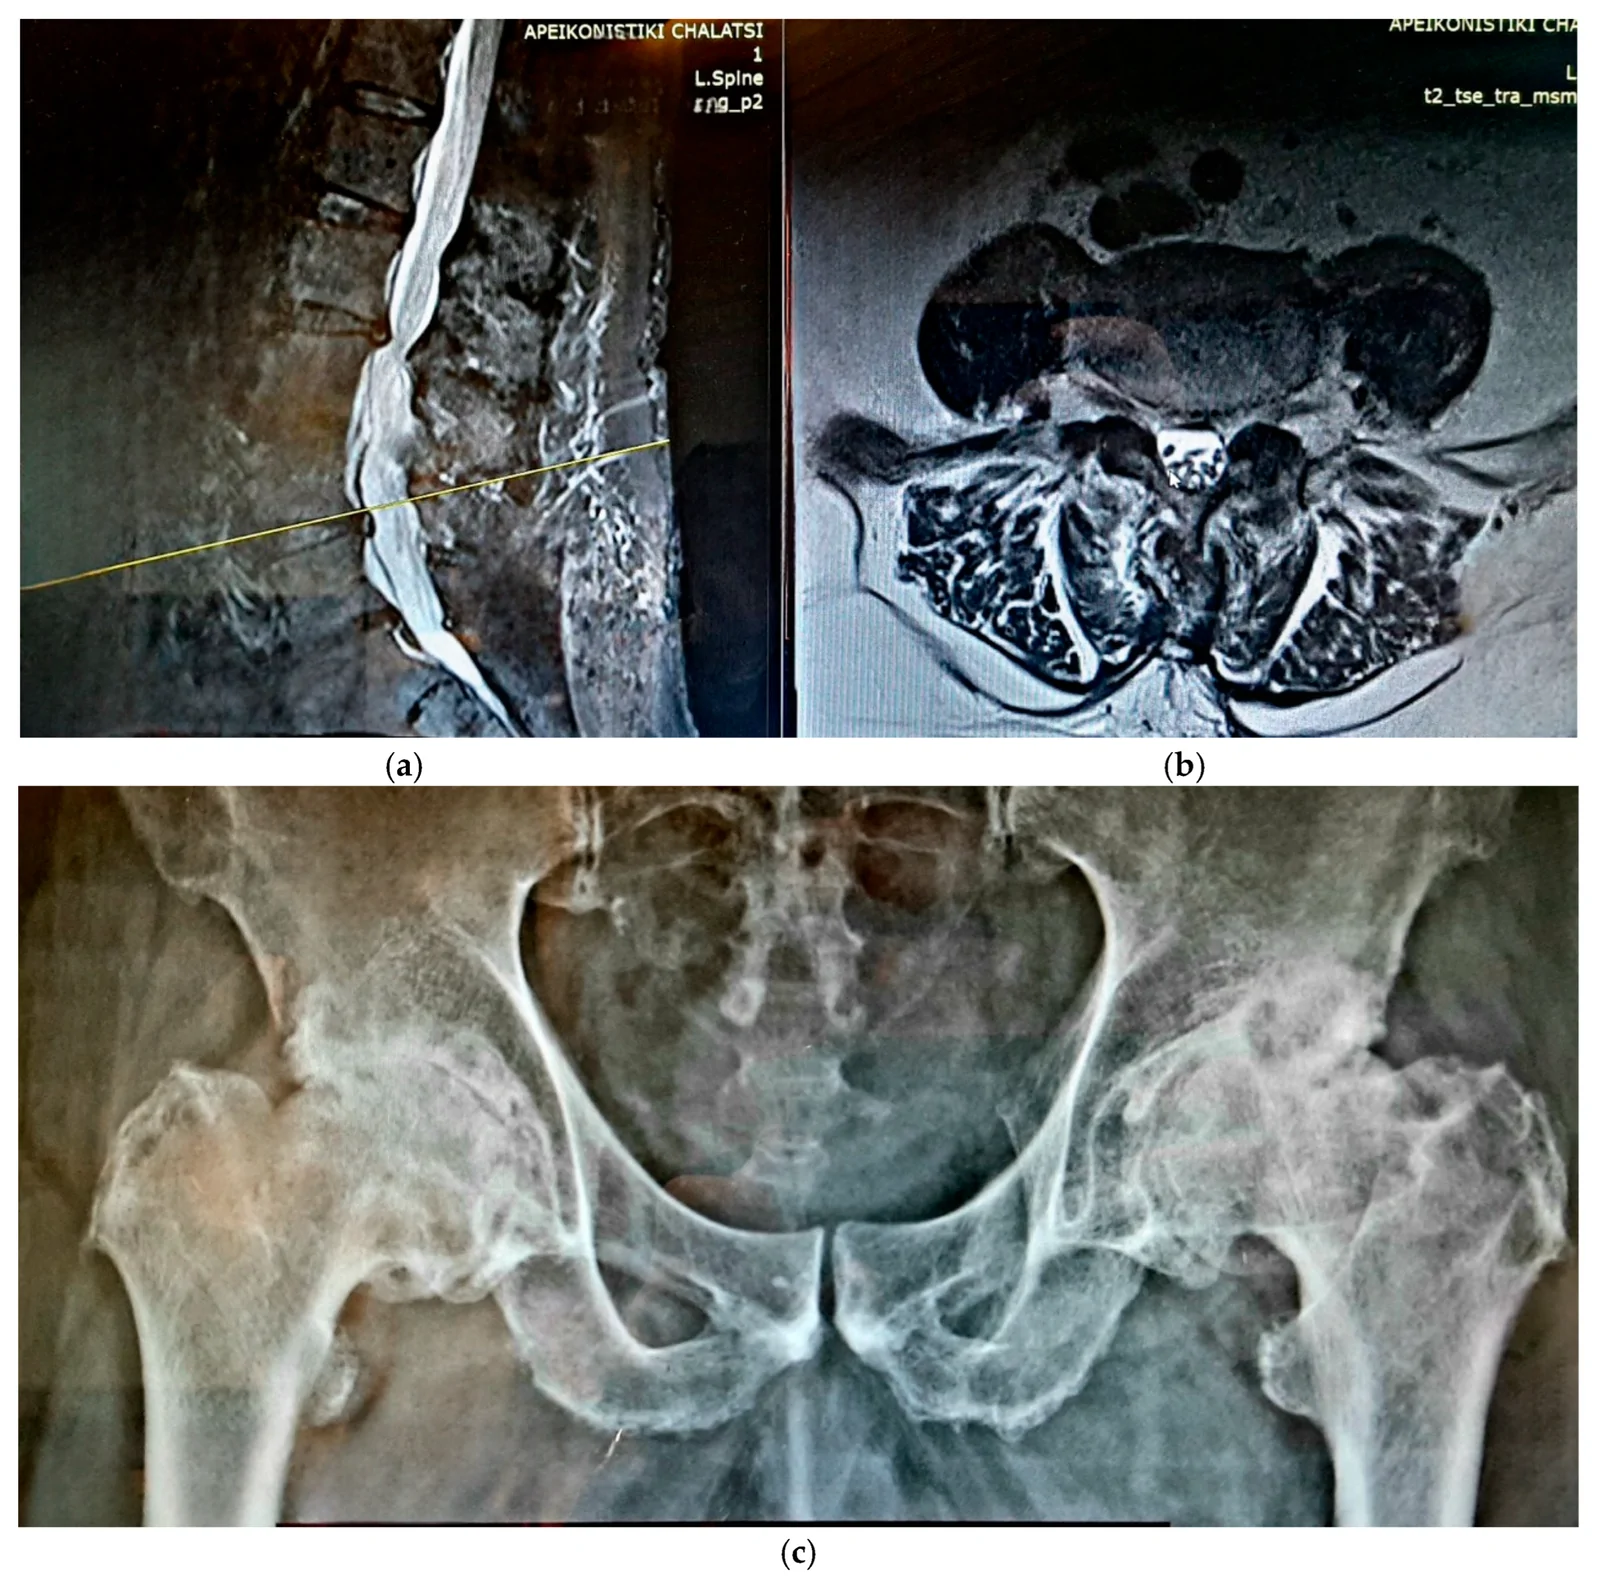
(a,b) MRI of the lumbar spine showing disc herniation in a patient who was surgically treated for sciatica symptoms. (c) X-ray of the hip demonstrating advanced osteoarthritis, which was the actual source of the pain, highlighting the need for differential diagnosis.
